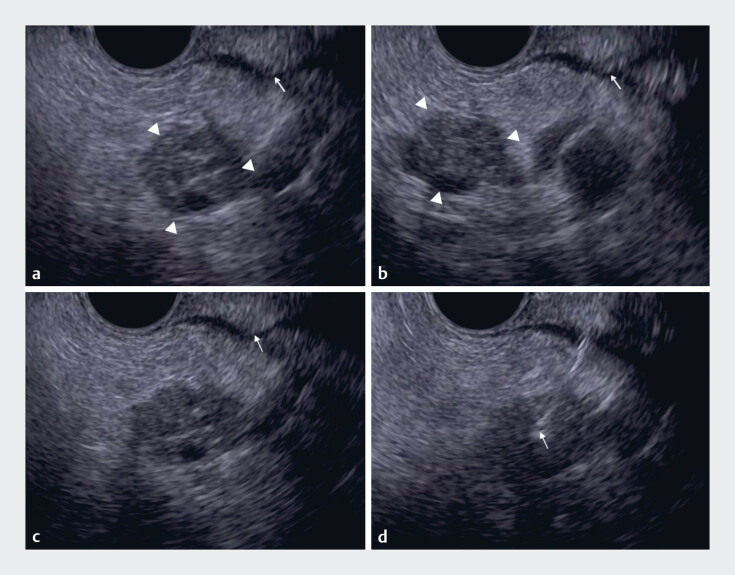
Needle loss from the endoscopic ultrasound (EUS) view due to axis misalignment of the endoscopy or external sheath.
